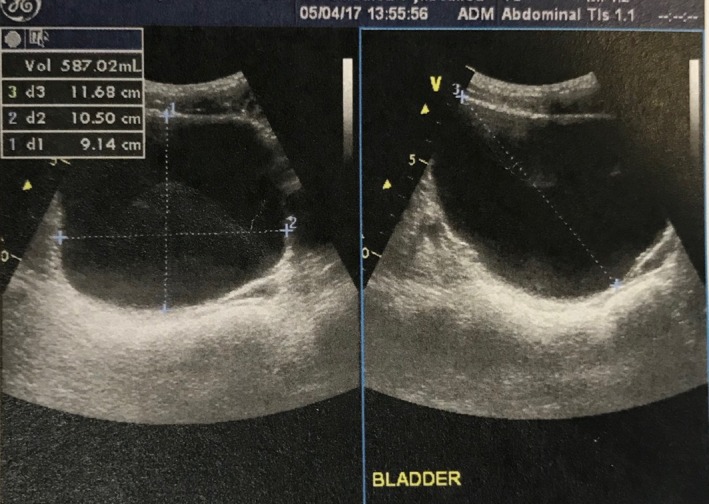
Image of transabdominal ultrasound of the bladder, failing to demonstrate intravesical papillary mass
